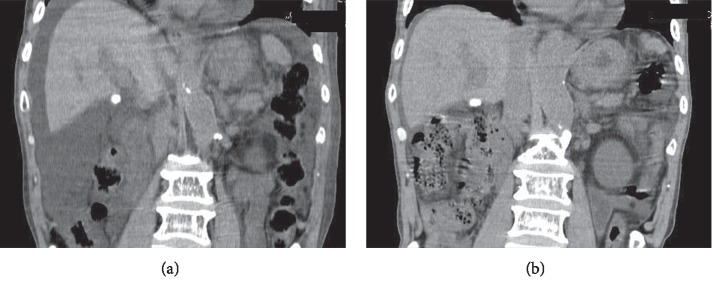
Abdominal CT showed massive storage of ascites (a) and a decrease in ascites after treatment (b).
